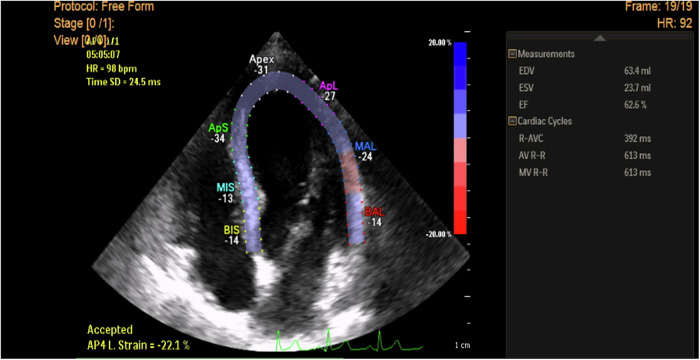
2D STE measurement of longitudinal strain in apical 4-ch view in a hypertensive patient without LVH shows a reduction of peak LS of basal segments of septal &lateral walls (Esys = −14%) but normal peak longitudinal strain of the remaining myocardial segments and normal global LV strain (GS = - 22.1%).
